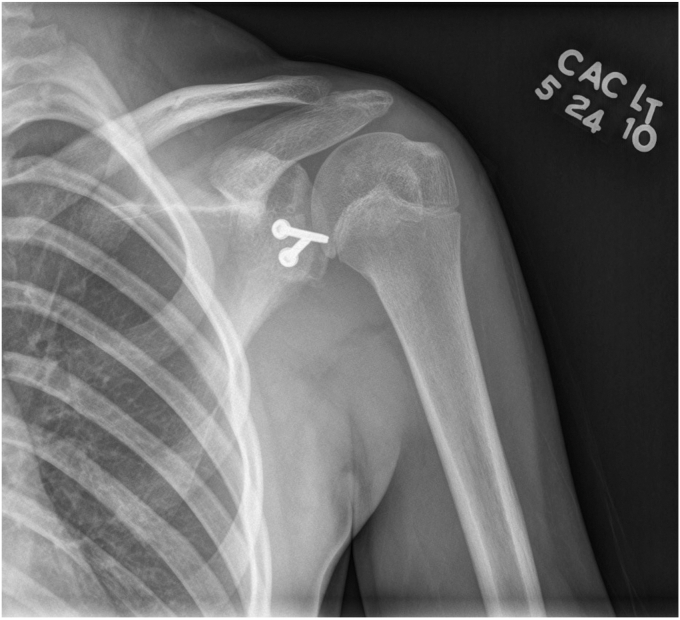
Anterior to posterior X-ray from 4.5 weeks after Latarjet.
